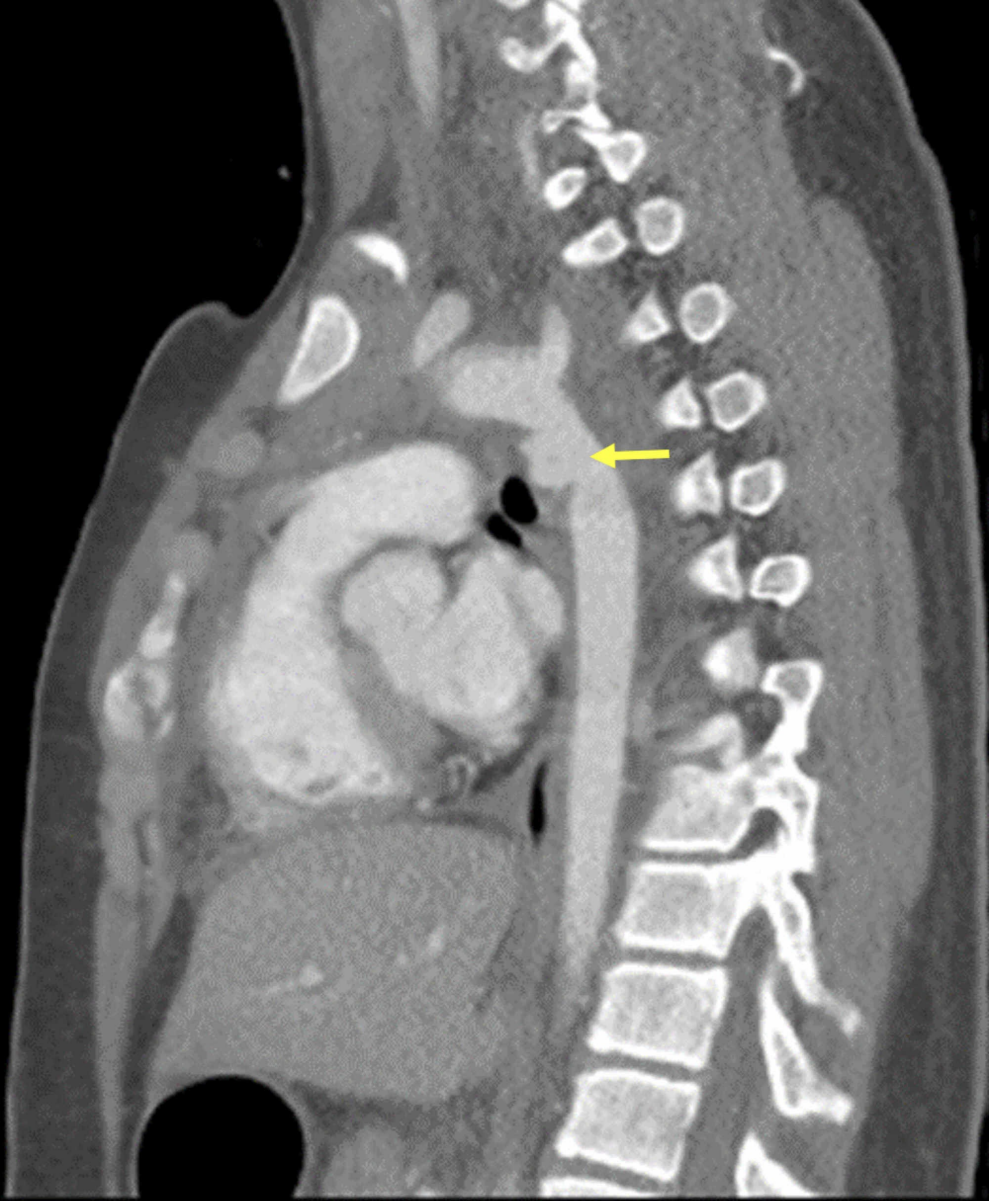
CT of the patient showing the pseudoaneurysm (yellow arrow) CT: computed tomography
